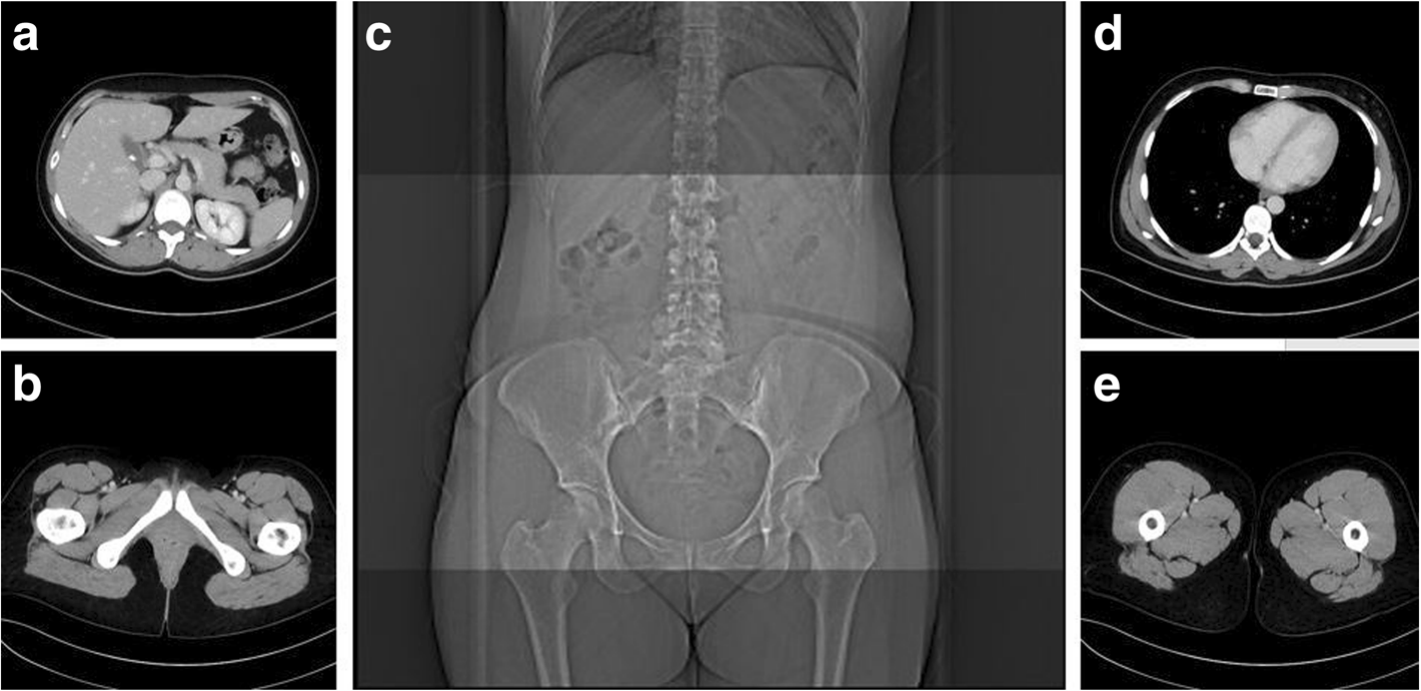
Axial reconstructed images displaying the upper and lower border of reduced range CT (a and b) as defined by the top of L1 and the pubic symphysis (c) compared to those of full range CT (d and e)
